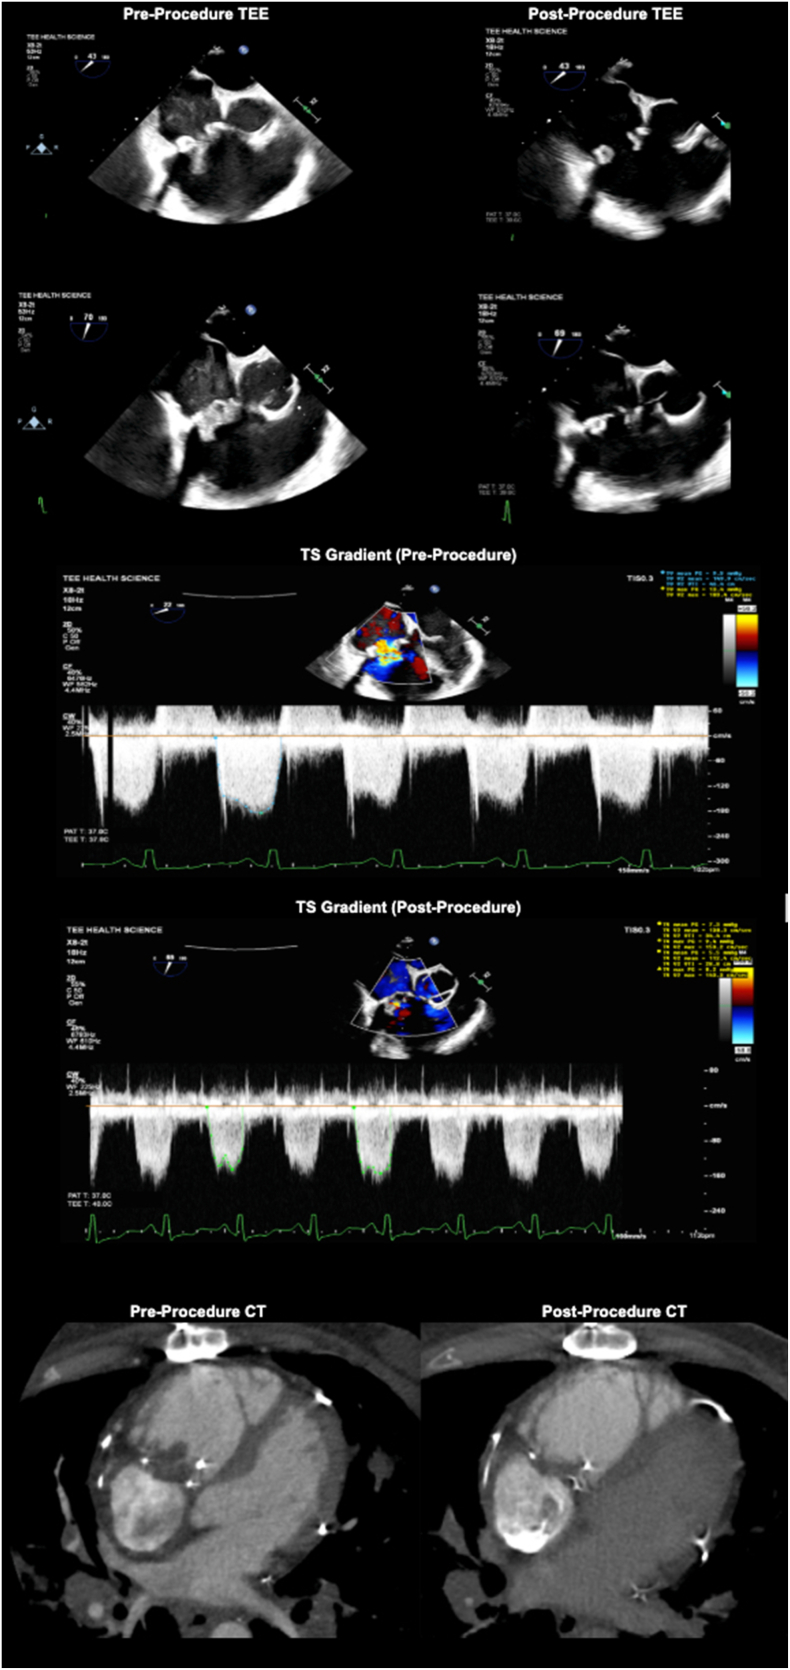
Top panels: Pre- and post-procedure transesophageal echocardiography (TEE) visualizations showing reduced mobility and size of the vegetation on the bioprosthetic tricuspid valve. Middle panels: Continuous wave Doppler. Bottom panels: Computed tomography (CT) demonstrating the vegetation burden (left) before and (right) after percutaneous mechanical aspiration. TS, tricuspid stenosis.
